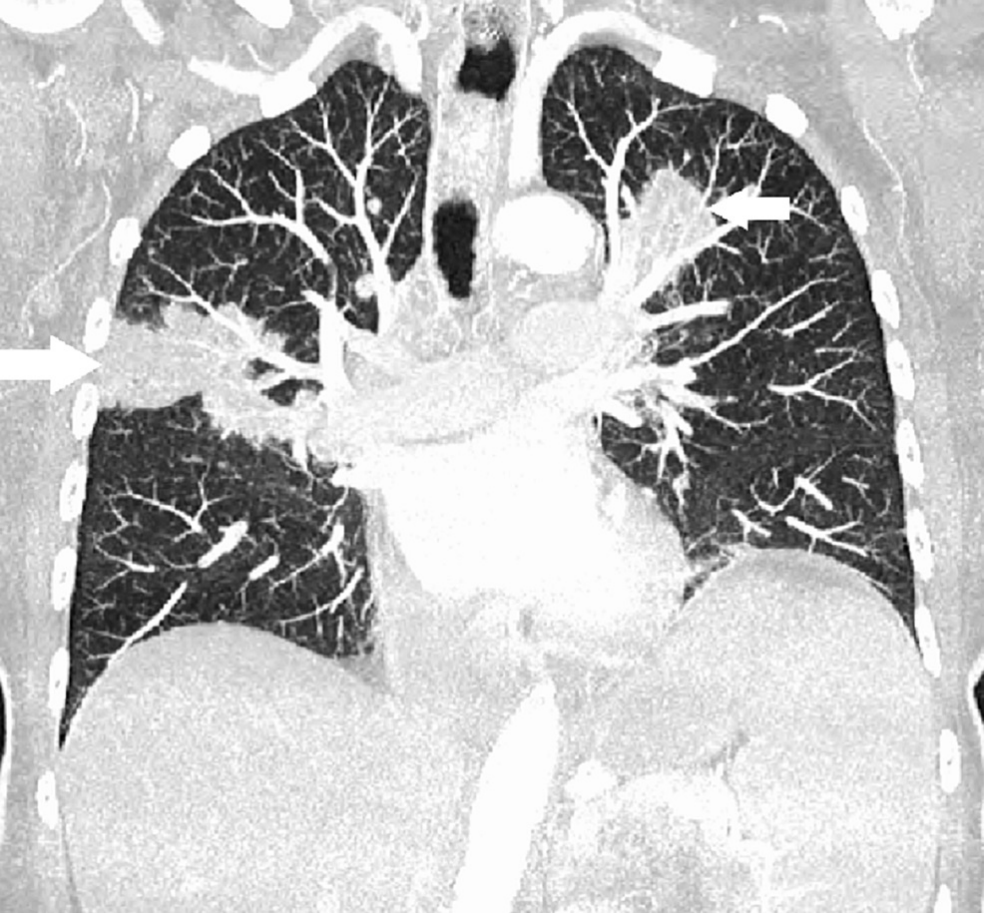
Coronal slice of CT chest indicating the bilateral pulmonary lesions/infiltrates (white arrows)
